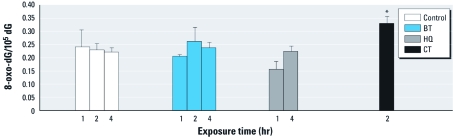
8-oxo-dG levels (expressed as the molar ratio of 8-oxo-dG per 10^5^ dG) in control HL-60 cells or cells exposed to 50 μM BT, 20 μM HQ, or 20 μM CT for 1, 2, or 4 hr; data presented are mean + SE of four independent experiments. p* < 0.05 compared with in corresponding control.
